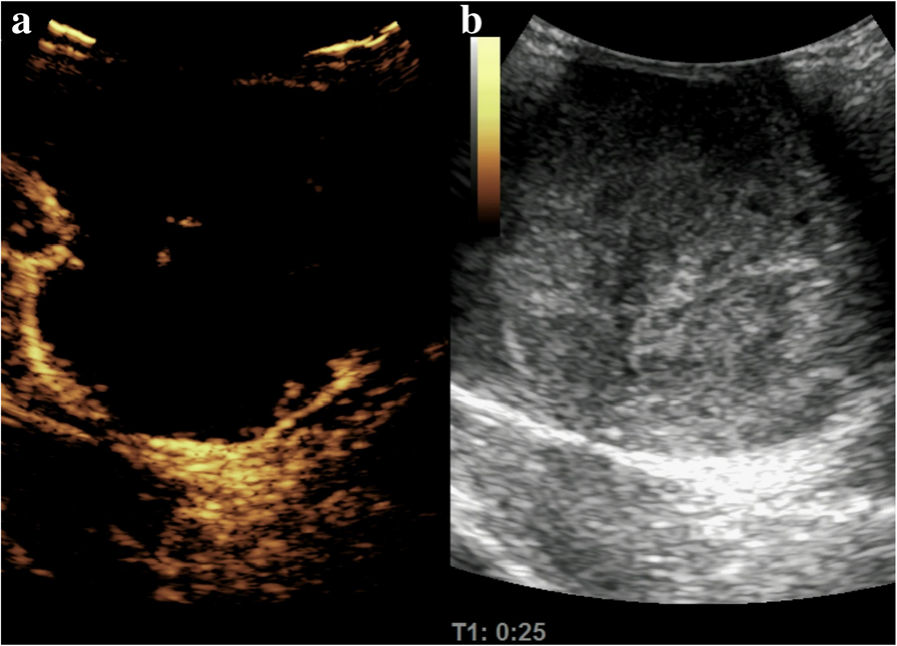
a Contrast-enhanced Ultrasonographic images showing that the mass enhanced from the periphery at 25 seconds after the bolus injection of SonoVue contrast agent. The coarse nourishing blood vessels are clearly displayed. b The corresponding two-dimensional ultrasound shows the morphologically abnormal right testis with heterogeneous internal echoes
